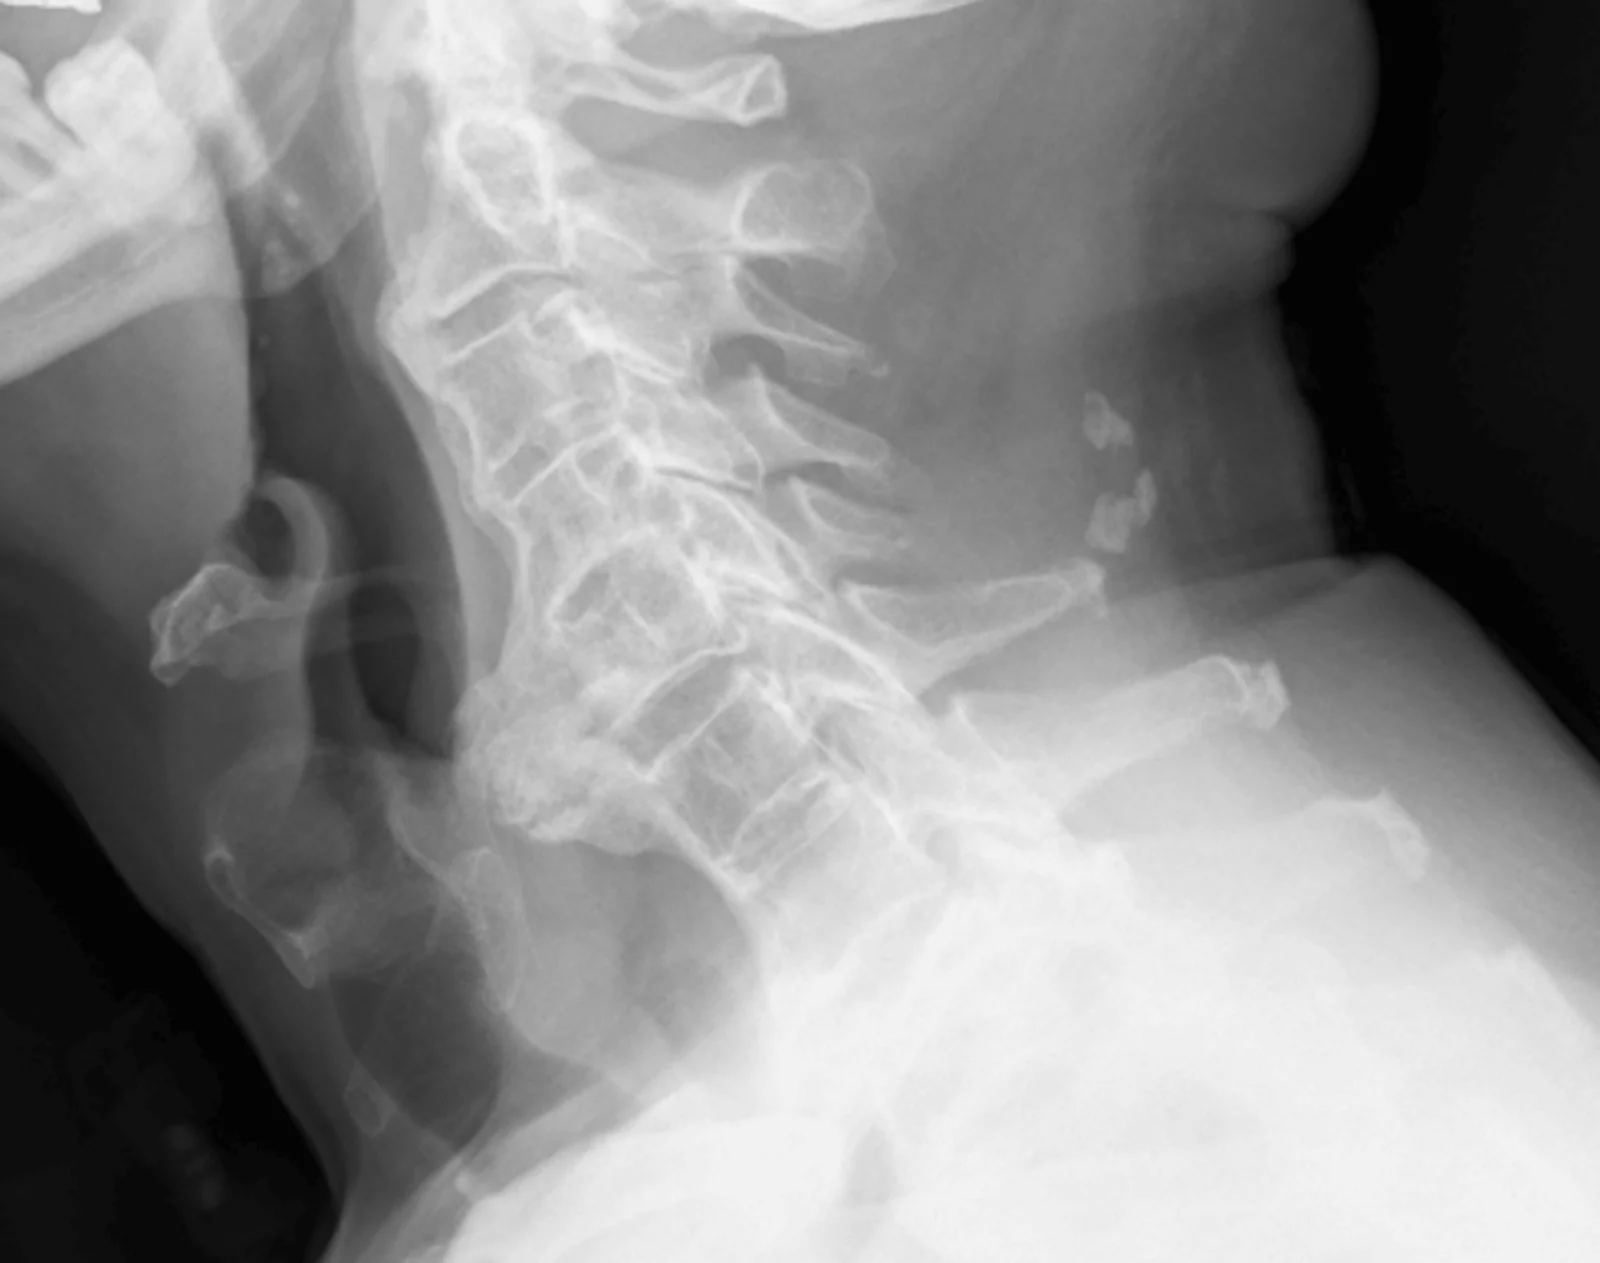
Lateral Cervical Spine Radiograph Lateral radiograph of the cervical spine demonstrating prominent, flowing anterior ossification along the anterolateral aspect of multiple contiguous vertebral bodies, consistent with ossification of the anterior longitudinal ligament. Intervertebral disc spaces are relatively preserved, and there is no radiographic evidence of significant apophyseal joint ankylosis.
